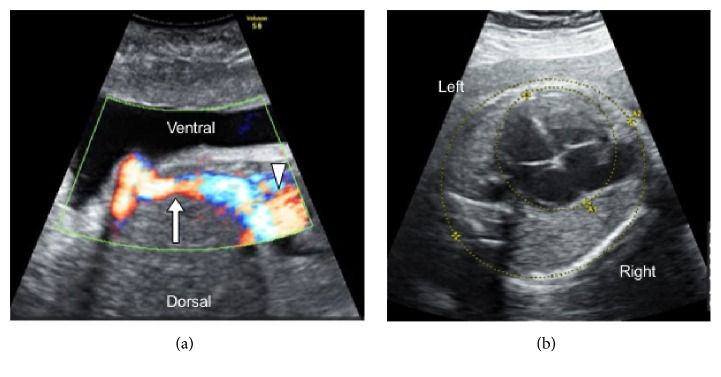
Prenatal ultrasound findings of a patient with absent ductus venosus. (a) The umbilical vein (arrow) directly flows into the right atrium (arrowhead). (b) The cardiothoracic area ratio was 36.5%.
